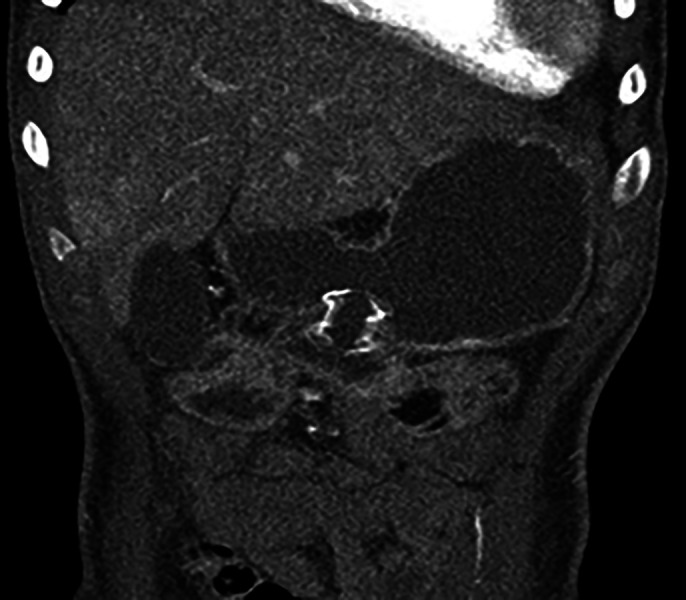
Abdominal computed tomography scan showing the gastrojejunal lumen-apposing metal stent.
